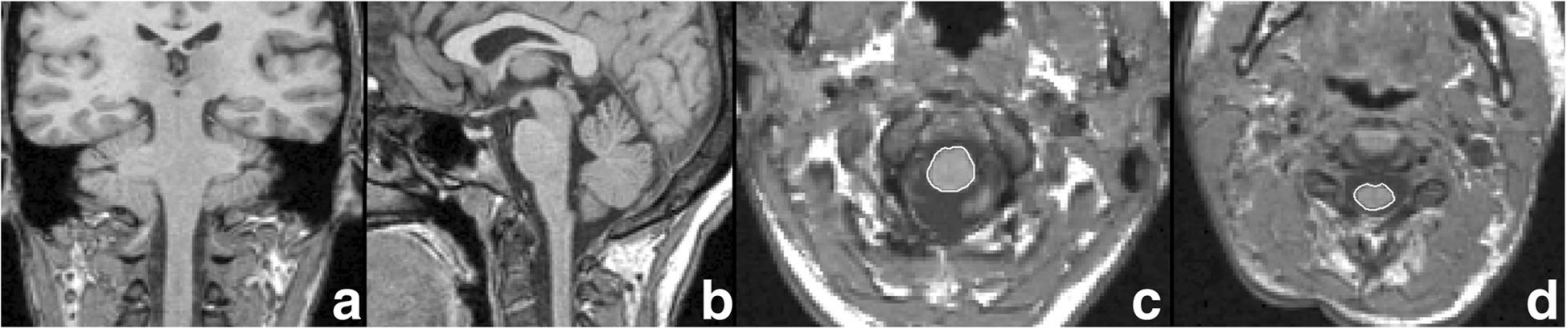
a and b Three-dimensional T1-weighted images volume-realigned perpendicularly to the main axis of the cord. Re-oriented axial views at the level of the foramen magnum (c) and at the C2-C3 level (d) depict the medians of five regions of interest for the quantification of cord area
